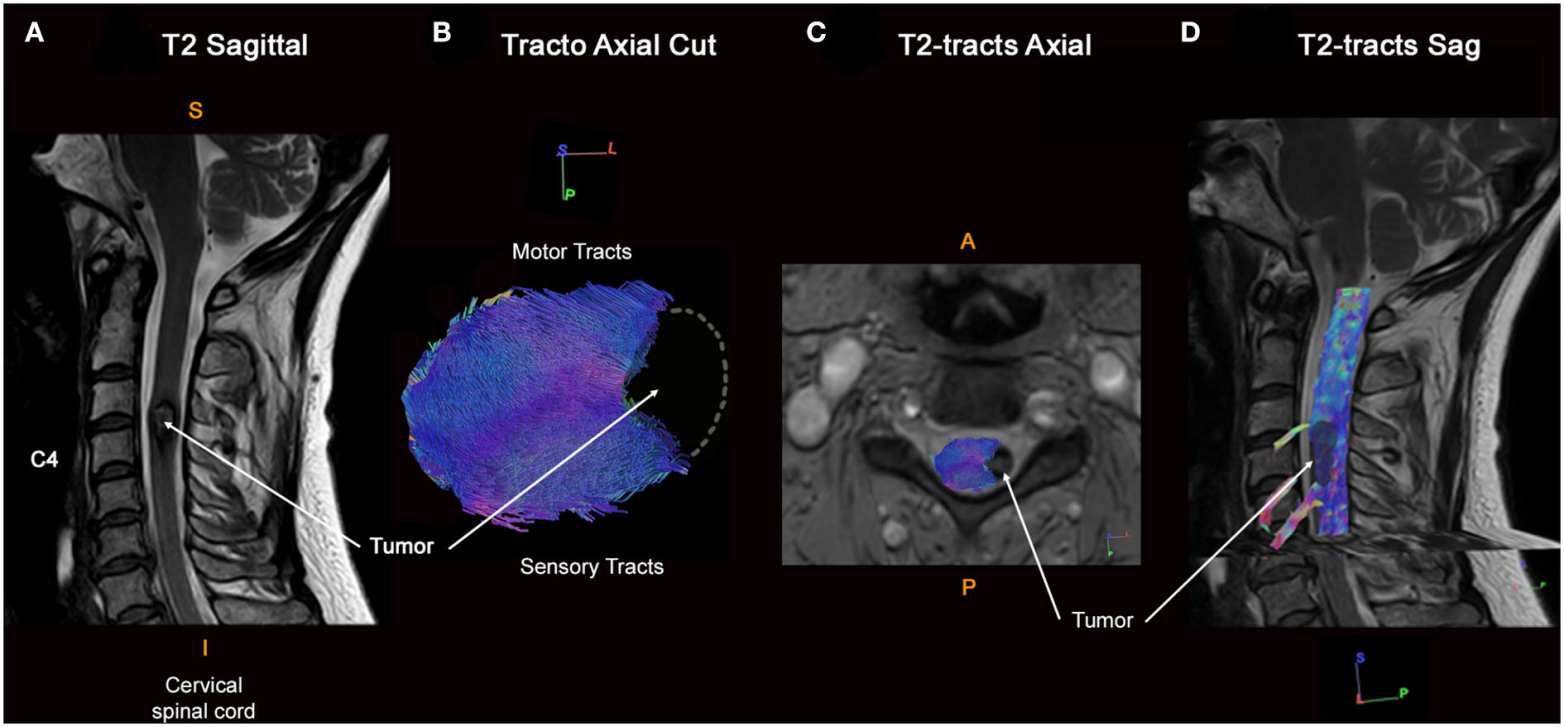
Full cervical cord tractography in a patient with intramedullary cavernous malformation. (A) Sagittal T2-weighed images showing the cavernous malformation at the C3–C4 spine level. Cavernous malformation presented an exophytic portion on the left side of the spinal cord, with a hemosiderin crown in a hyposignal T2-weighted sequence. (B) Axial view of spinal cord tractography at the C3–C4 level shows that spinal fibers were pushed back and compressed by the cavernous malformation, without fibers around the cavernous malformation (at its dorso-lateral part). Left lateral view of cervical spinal cord tractography showing a notch within the spinal cord repressing spinal tracts. (C) Overlay of the spinal cord tractography on the axial T2-weighted MR images. (D) Overlay of the spinal cord tractography on the sagittal T2-weighted MR images, with the 3D rendering of the cavernous malformation (in black).
